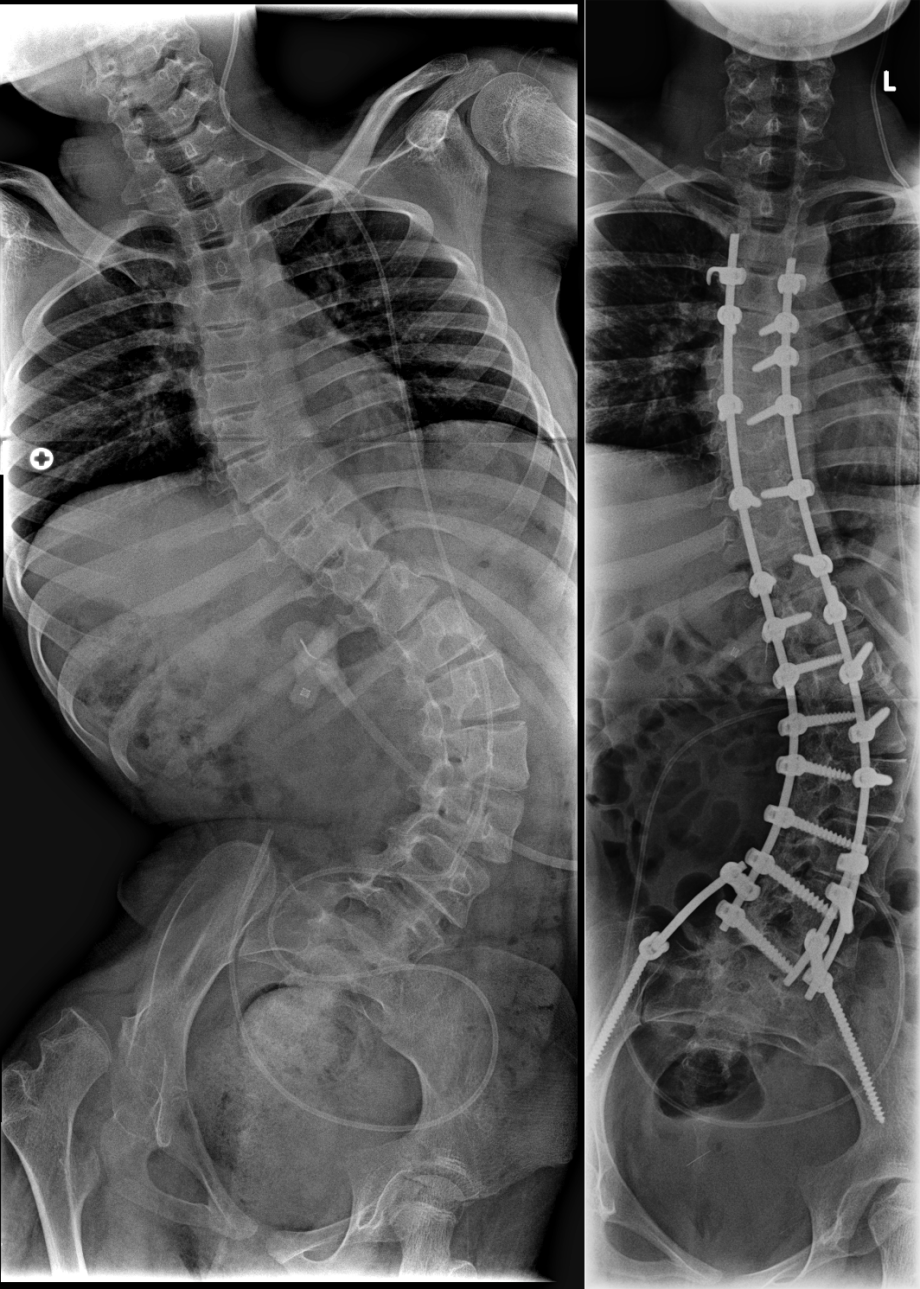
Case 1. Anteroposterior radiographs made preoperatively (Figure 2a) and following posterior instrumentation from T3 to the pelvis (Figure 2b) in a 8-year old patient with a severe neuromuscular scoliosis.
